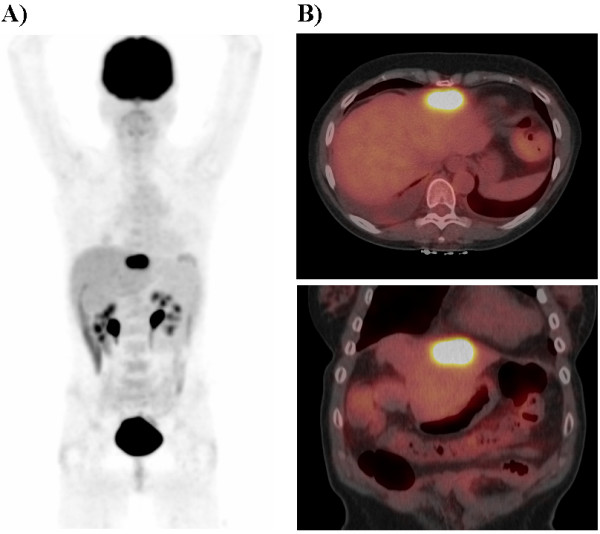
Preoperative PET maximum intensity projection in the anterior projection view (A) and cross sectional fused PET/CT images (B). The preoperative PET/CT scan revealed a solitary hypermetabolic focus in the left lobe of the liver.
